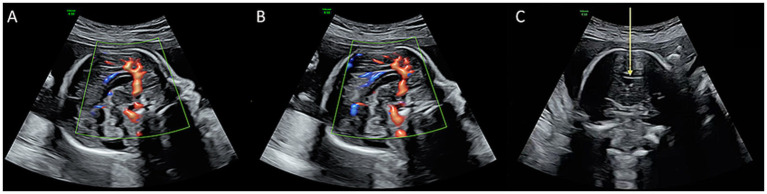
Transcranial ultrasound imaging of the neonatal corpus callosum. (A) Echogenicity of the corpus callosum on a normal midsagittal two-dimensional cranial section. (B) Color Doppler imaging demonstrating the pericallosal artery (PA) running along the superior margin of the corpus callosum. (C) Coronal section showing corpus callosum echogenicity. The arrow indicates the level of the anterior horns of the lateral ventricles on both sides of the frontal lobe. By angling the sound beam approximately 10°, the anterior horns of the lateral ventricles, cavum septum pellucidum (CSP), corpus callosum (seen as a thin, arc-shaped hypoechoic band above the CSP), and the foramen of Monro (interventricular foramen) are visualized. CC, corpus callosum; CSP, cavum septum pellucidum; 3V, third ventricle.
